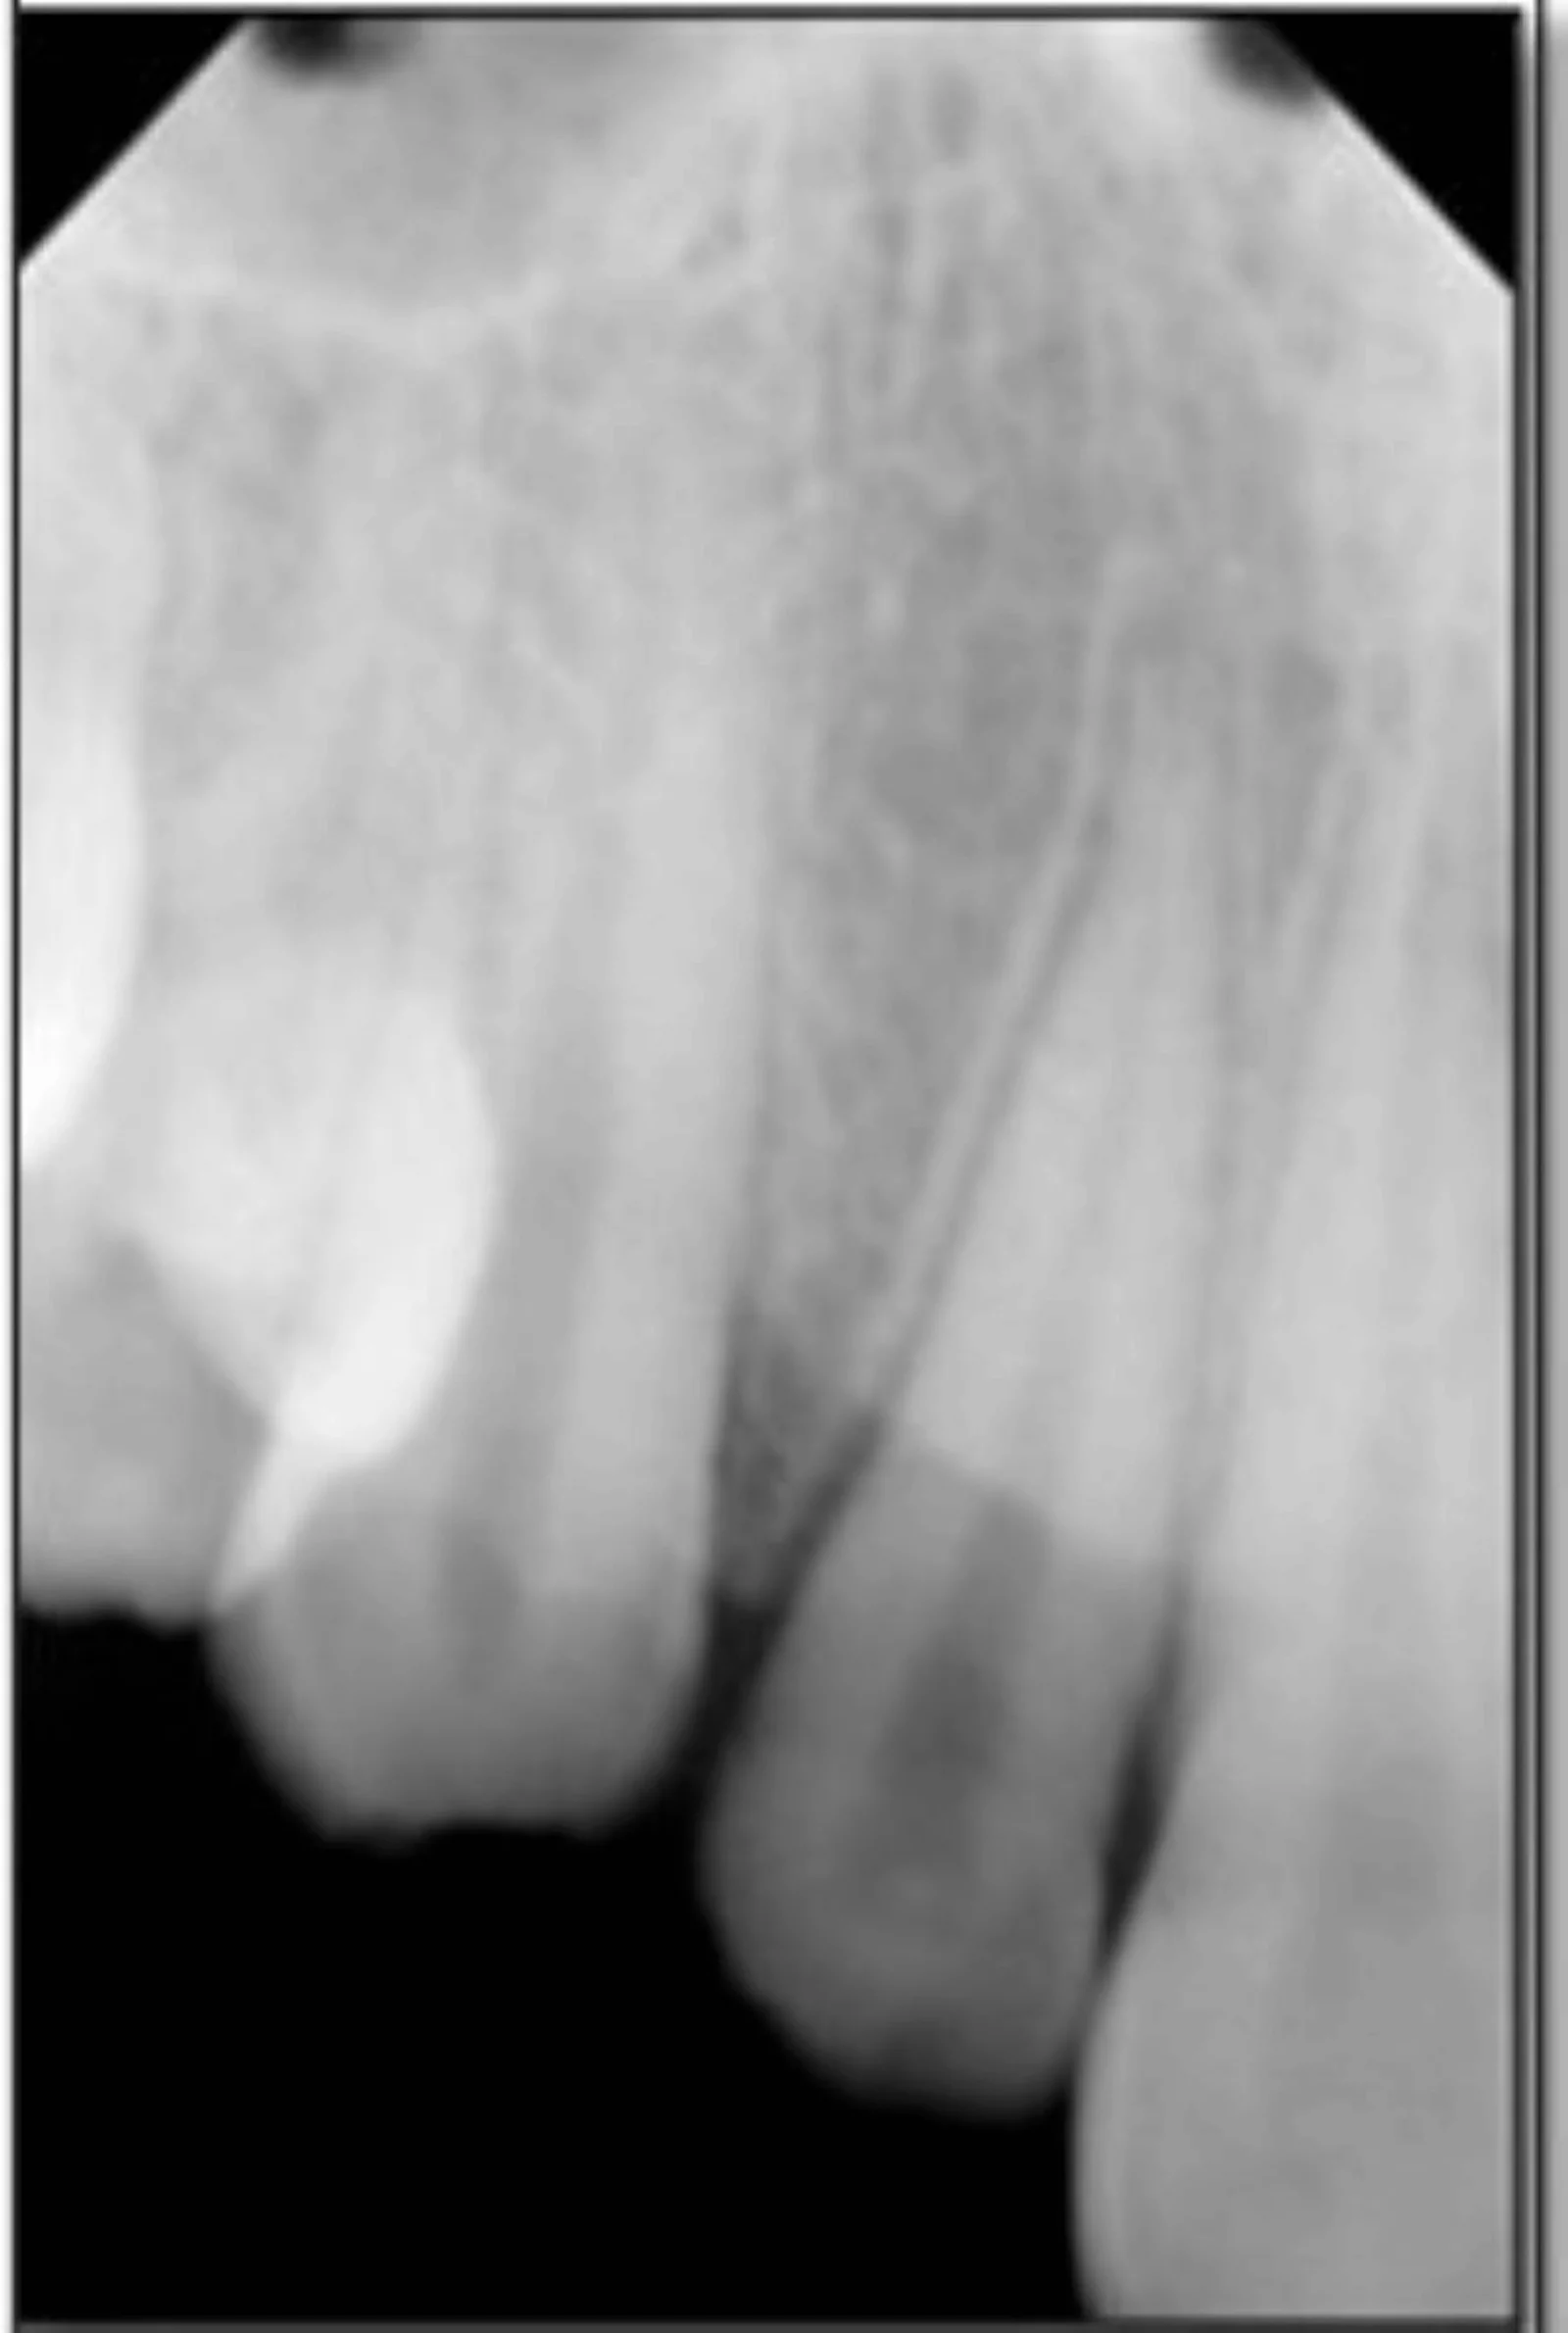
Intraoral periapical radiograph demonstrating widening of the periodontal ligament space and a closed root apex.
